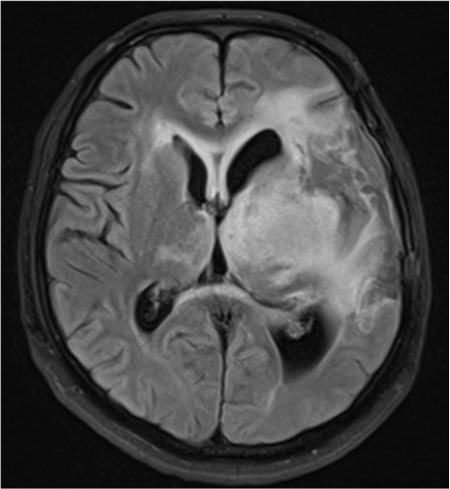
The extent of the new lesions in the left hemisphere (frontal, temporal, parietal region) was demonstrated by FLAIR MRI sequence with subependymal infiltration crossing to the right hemisphere
